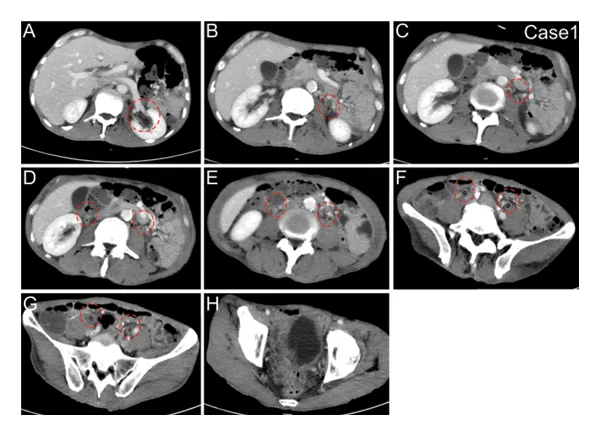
Case 1 patient’s CT scan revealed the inflammation of bilateral ureteral and bladder wall. The abdominal CT revealed mild bilateral hydronephrosis with mural thickening and enhancement of both ureters and the bladder, findings consistent with inflammation.
